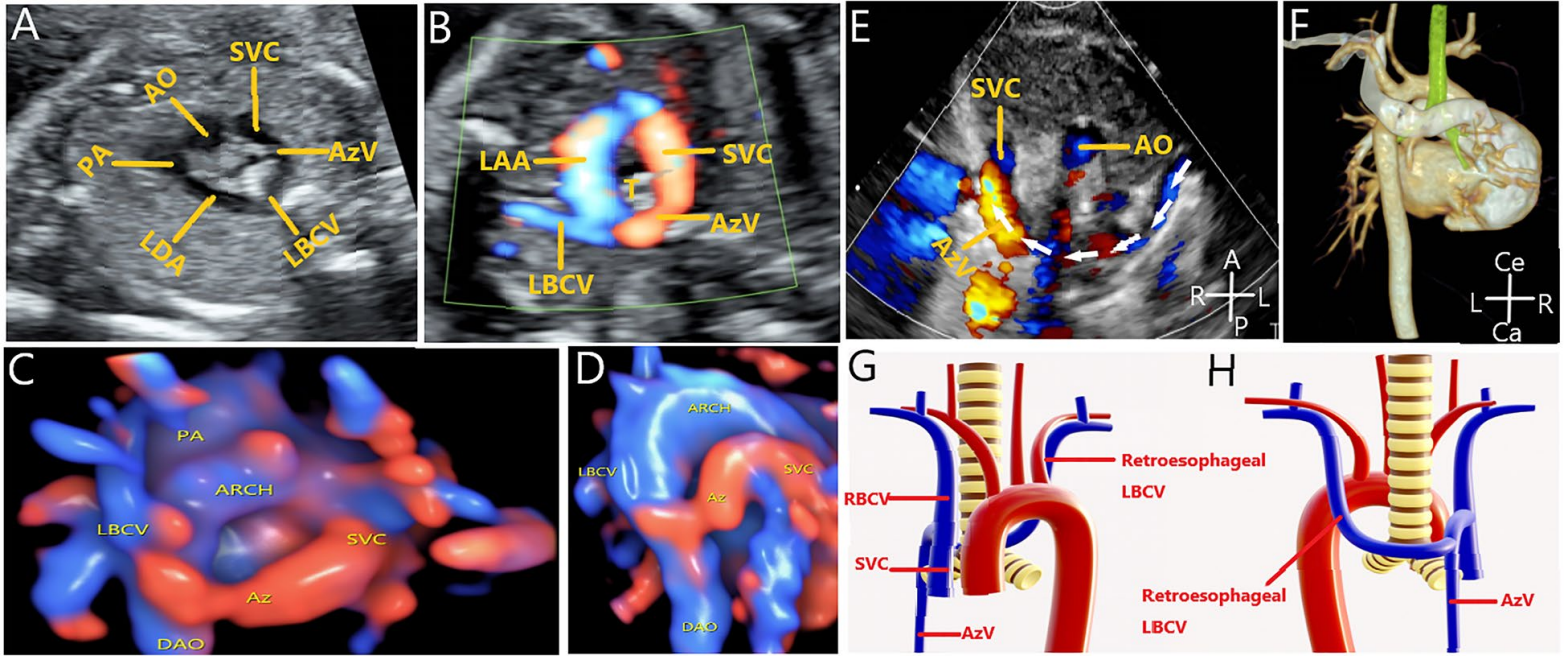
Image of the retroesophageal LBCV. A The LBCV runs behind the aorta and flows into the RSVC to form a U-shaped structure. B Color blood flow showing the retroesophageal LBCV. C–D Retroesophageal LBCV is shown using HD-flow render mode and STIC. E Postpartum 2D ultrasound image showing that the LBCV bypasses the esophagus. F Postpartum CTA showing that the LBCV bypasses the esophagus and merges into the RSVC, which was consistent with the prenatal image. G-H Structural presentation of a retroesophageal LBCV. G Anterior view. H Posterior view. LBCV, left brachiocephalic vein. AO, aorta; PA, pulmonary artery; SVC, superior vena cava; LDA, left ductus arteriosus; AzV, azygos vein; LAA, left aortic arch; ARCH, aortic arch; T, trachea; DAO, descending aorta; RBCV, right brachiocephalic vein; L, left; R, right; Ce, cephalic; Ca, caudal; A, abdominal; P, spinal
